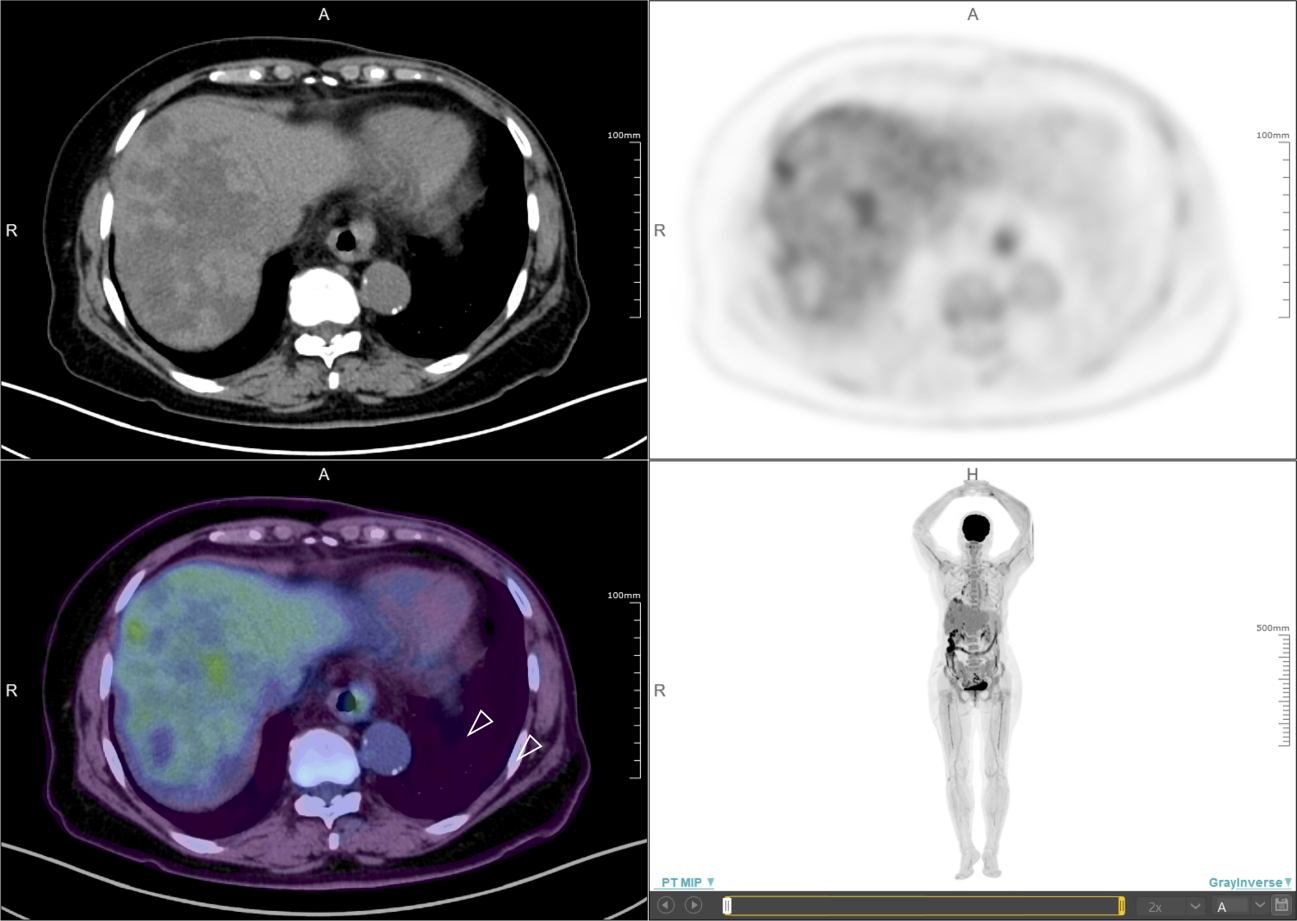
Positron emission tomography/computed tomography (PET/CT). PET/CT scan confirmed slightly high fluorodeoxyglucose (^18^F-FDG) uptake with an SUV_max_ of 5.9 for limited metastatic liver lesions (white arrow).
